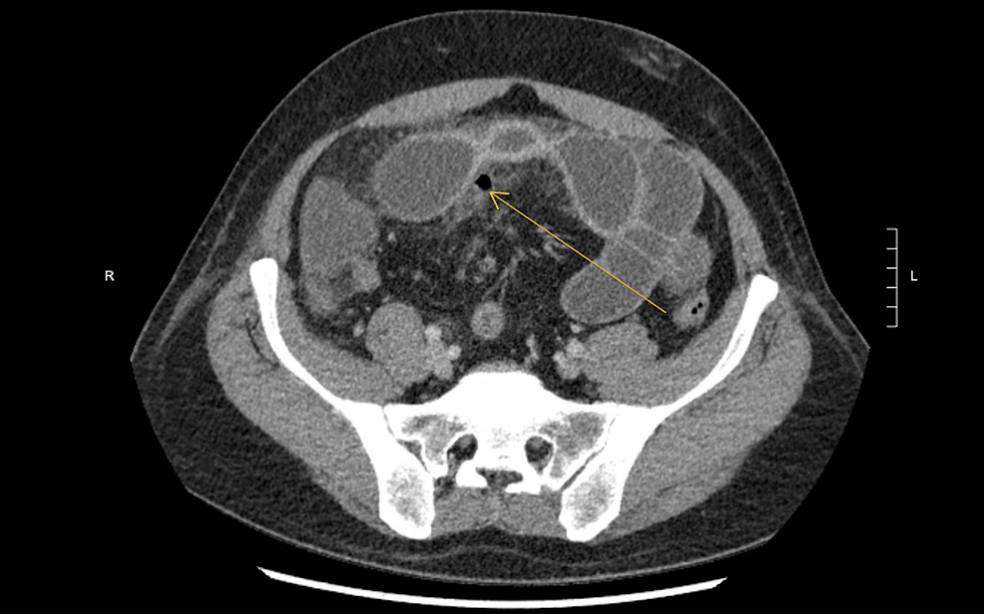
Computed tomography imaging shows worsening inflammation of the ileum with extraluminal air (arrow) and concern for obstruction.
